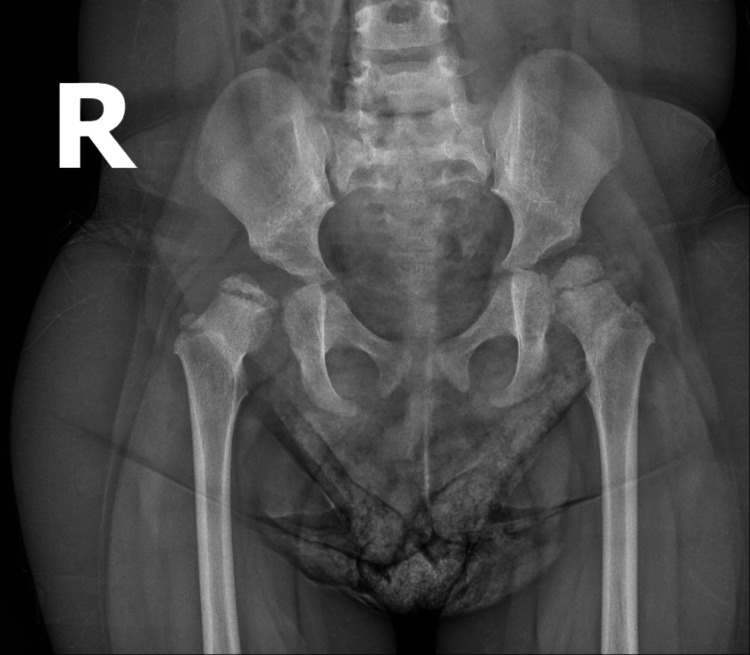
Two-year follow-up radiograph demonstrating hip subluxation, disrupted Shenton-Menard line, and persistent acetabular dysplasia
